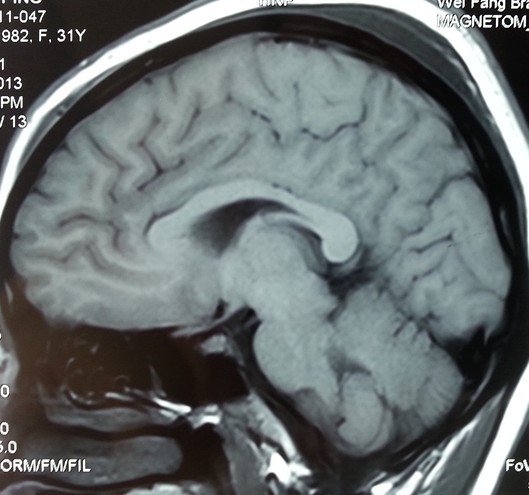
Postoperative magnetic resonance imaging of the brain. Postoperative cranial magnetic resonance imaging revealed satisfactory result.
